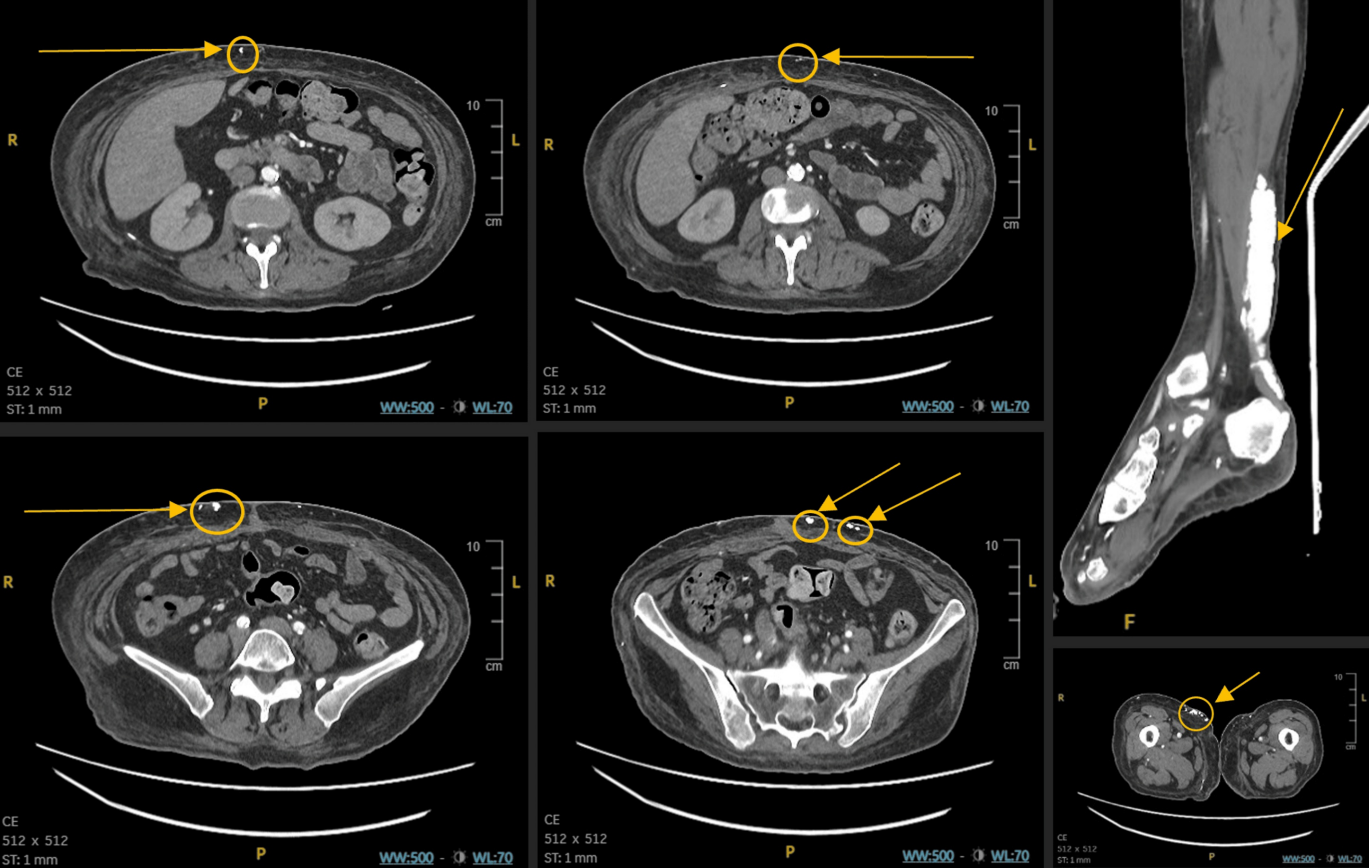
CT angiography of the lower limbs showing subcutaneous and intramuscular calcification. Please note the extensive calcification of the Achilles tendon.
